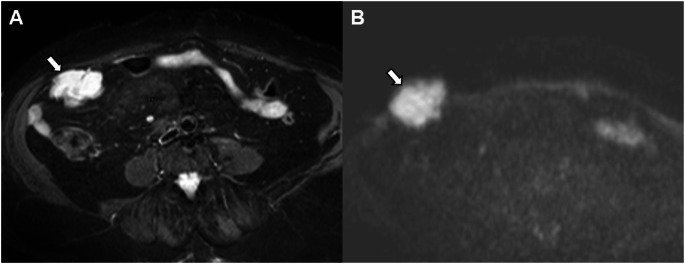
Extended MR study of the abdomen in a 65-year-old woman with uterine serous carcinoma. A Axial fat-suppressed T2-weighted fast spin-echo (FSE) image (TR/TE: 4500/100 ms; slice thickness: 3 mm) and B diffusion-weighted image (DWI) with b = 1000 s/mm^2^ show a peritoneal implant beyond the pelvis (arrow). Pathological analysis confirmed metastasis
